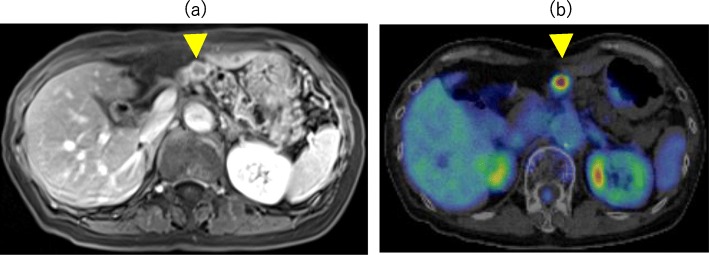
a Contrast enhanced MRI. revealing a peripherally-contrasted irregular tumor in the remnant pancreas (arrow head). b PET-CT. The maximal standardized uptake value (SUV max) was 6.8 at the tumor (arrow head)
